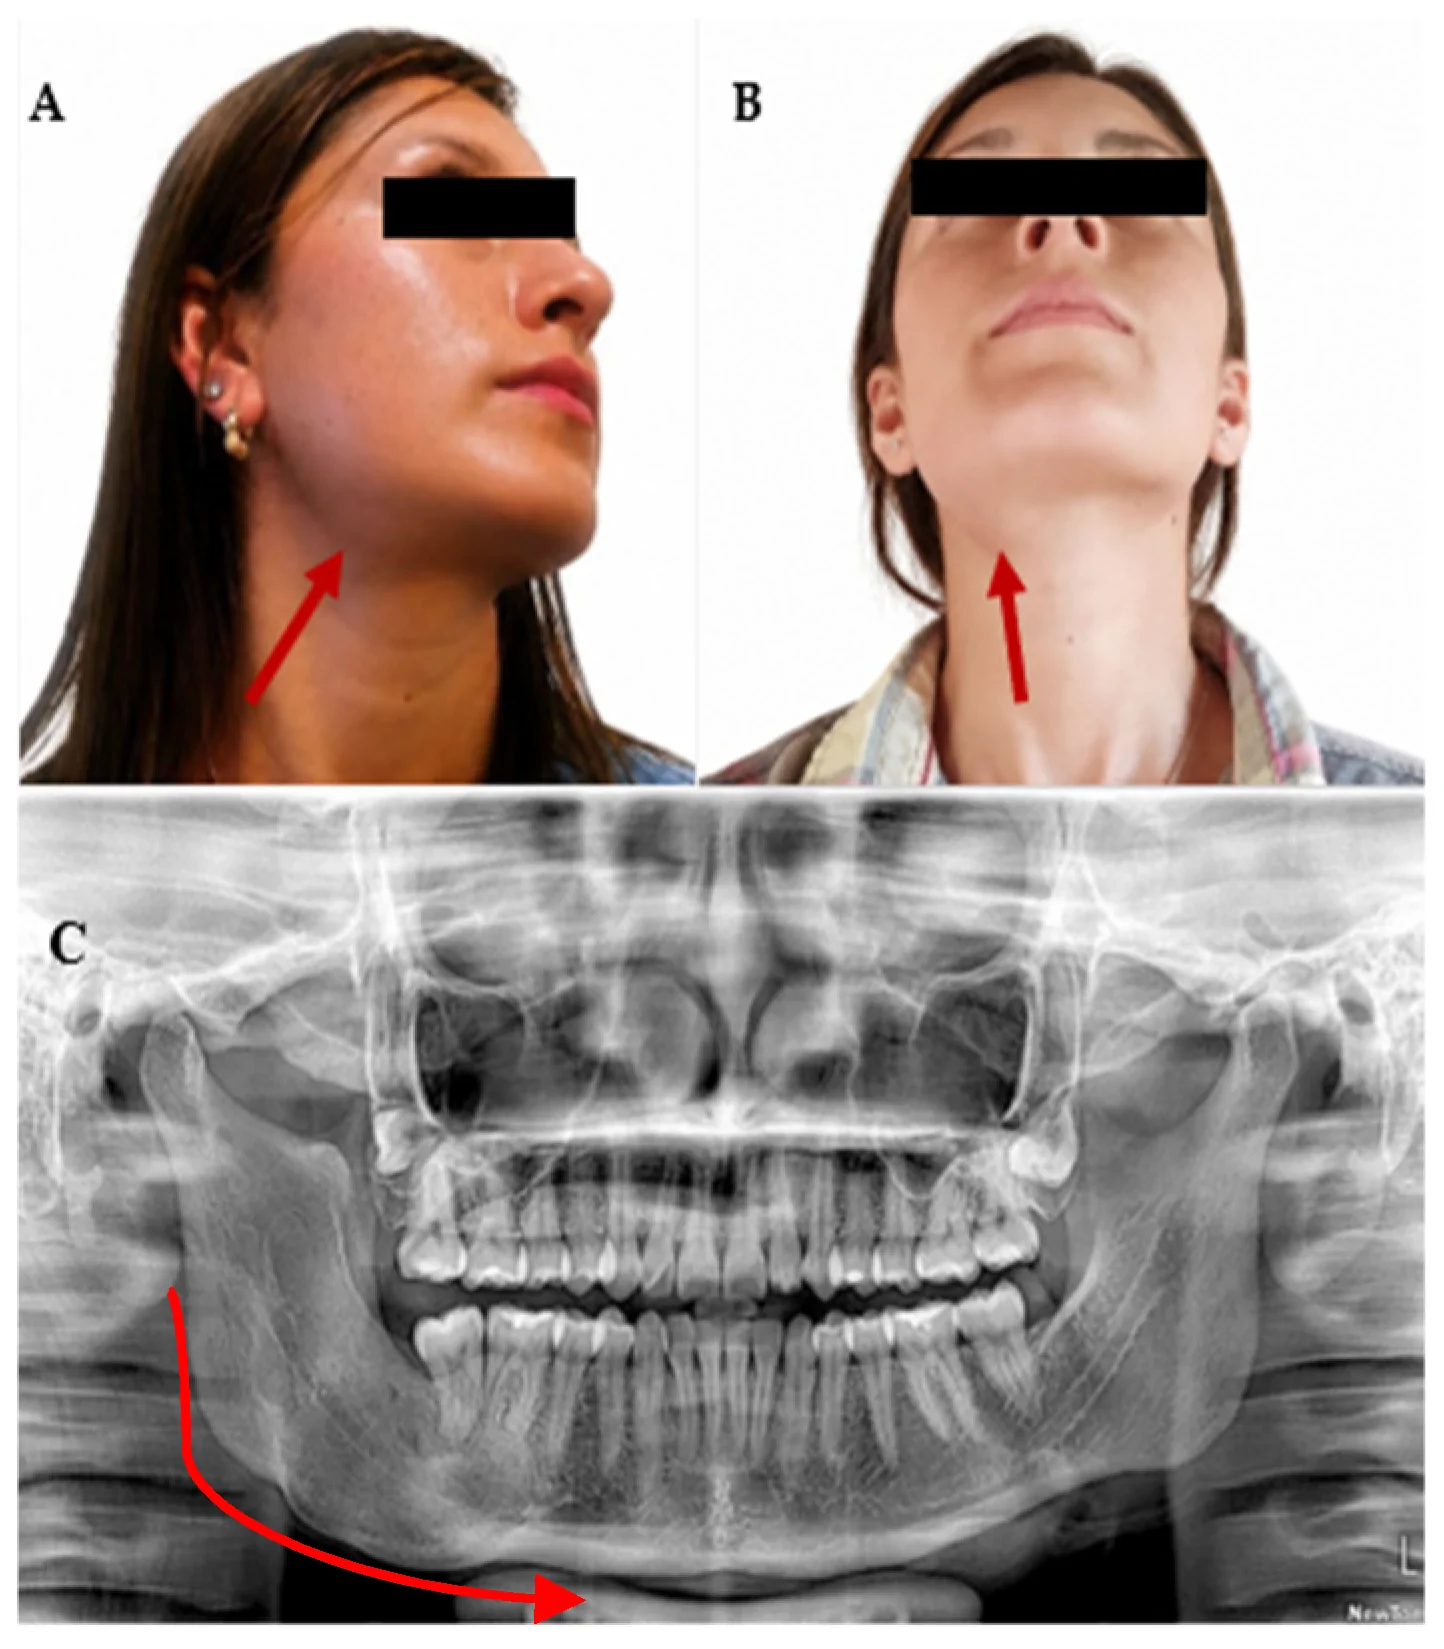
Clinical and radiographic follow-up at 1 year. (A) Lateral extraoral view. (B) Submental extraoral view. (C) Panoramic radiograph at 1-year follow-up. The red arrows indicate improved facial symmetry in the right submandibular region and restoration of anatomical contour.
